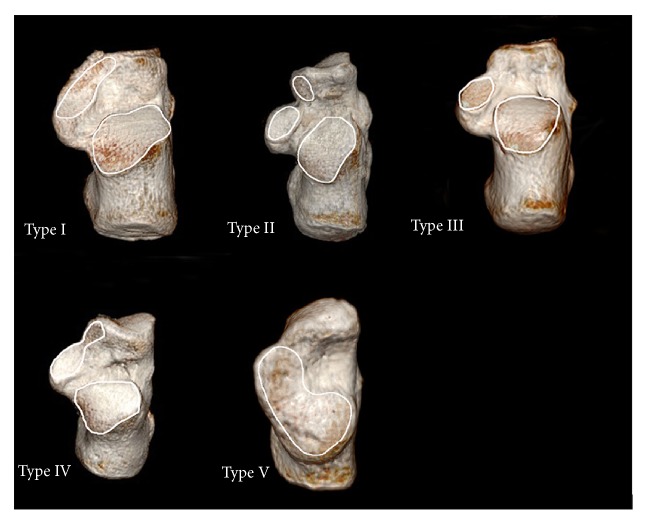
Three-dimensional CT reconstruction of calcaneal talar facet*. Type I: anterior and middle facets were fused into a pear-shaped big facet. Type II: three separate anterior, middle, and posterior facets. Type III: missing anterior facet. Type IV: anterior and middle calcaneus facets were fused into a calabash-shaped big facet. Type V: absence of the anterior facet, and the middle and posterior ones were fused.
